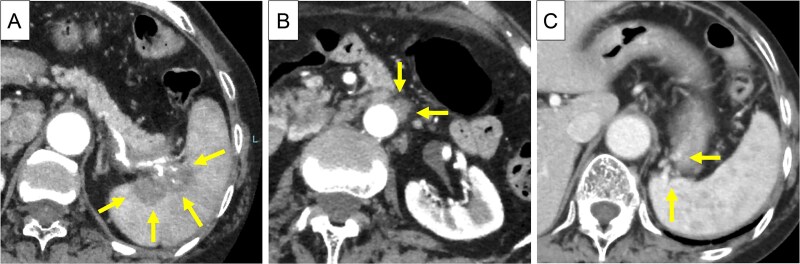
CE-CT before chemotherapy. (A) A 5 cm-sized neoplastic lesion in the pancreatic tail, with invasion into the splenic artery and splenic parenchyma (arrows). (B) the Para-aortic lymph nodes were enlarged (arrows). (C) Due to obstruction of the splenic vein at the hilum of the spleen, collateral veins had developed in the posterior wall of the stomach (arrows).
